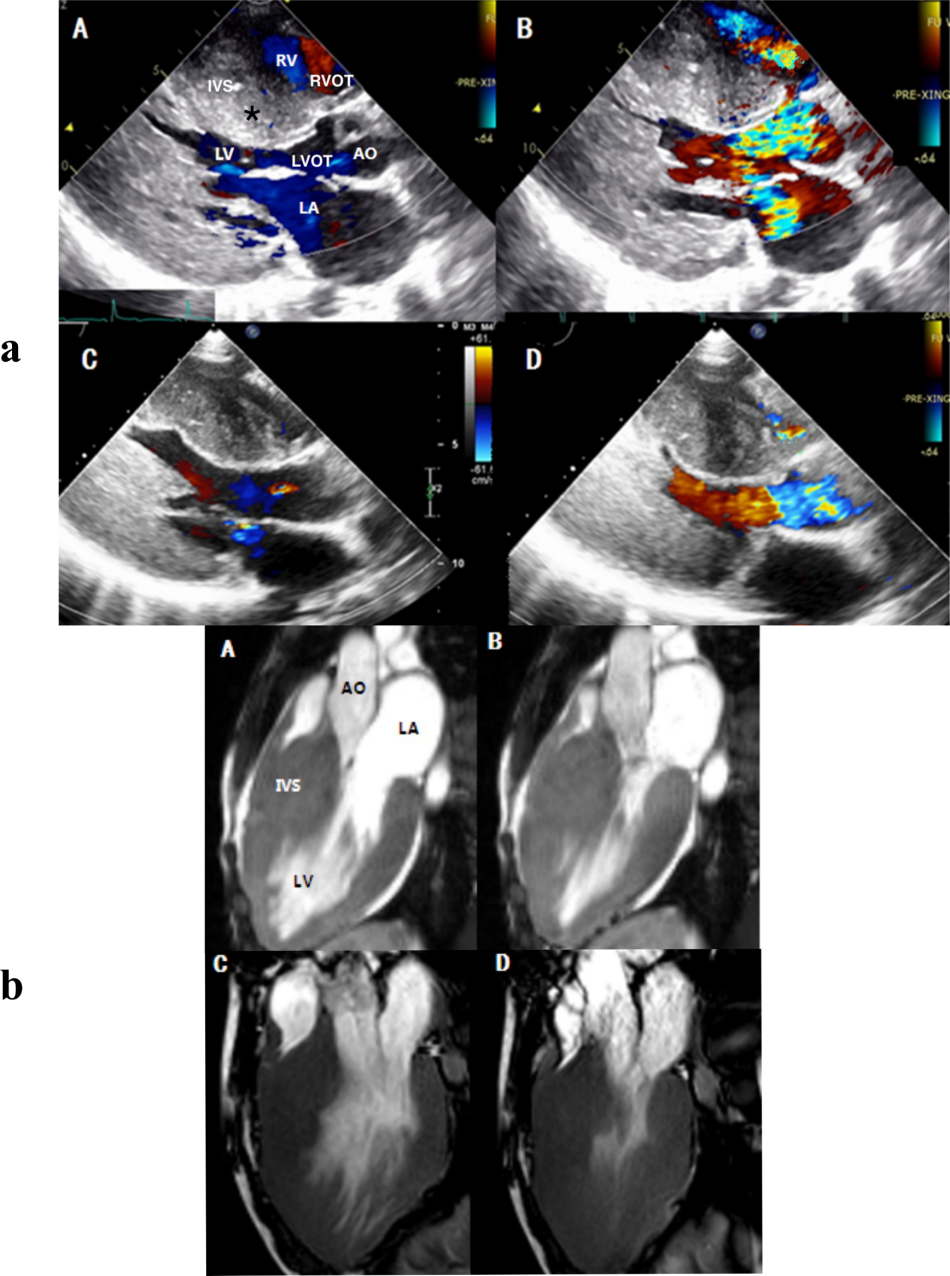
Fig 2a. Preoperative two-dimensional transthoracic echocardiography (tte) parasternal long axis (PLAX) views in a 16-year-old hypertrophic cardiomyopathy patient with BVOTO. (A) PLAX view demonstrating the massive septal hypertrophy and the thickening of the ventricular septum bulging into the LVOT and RVOT resulting in biventricular obstructions (the colour flows). (B) Colour Doppler flow imaging of PLAX view during systole showing high velocity jet flow simultaneously in both LVOT and RVOT. Postoperative PLAX views showing a substantial decrease in the ventricular septum thickness and an increase in the RV and LV cavity sizes during diastole (C) and the LV and RV colour flows showing laminar without evidence of significant residual obstructions during systole (D).RV: right ventricle; RVOT: right ventricular outflow tract; IVS: interventricular septum; LV: left ventricle; LA: left atrium; LVOT: left ventricular outflow tract.AO: aorta. Fig 2b. Preoperational cardiovascular magnetic resonance (CMR) image 3-chamber views during diastole (A) and systole (B) showing remarkable myocardial hypertrophy at the base ventricular level with LVOT and RVOT obstruction. The postoperative CMR images (C, D) showing thinner IVS, wider LVOT and RVOT diameter and larger LV and RV cavity without the projection of septum into RVOT or LVOT after biventricular resection. LA: left atrial; LV: left ventricular.
